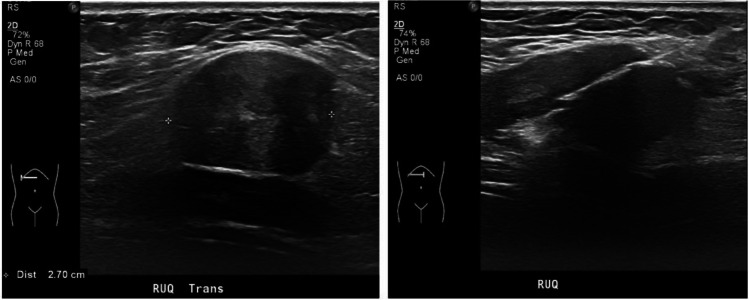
Ultrasound biopsy of the right upper abdominal wall mass. The mass was perceived as very firm or dense, providing high resistance to penetration with the biopsy needle
